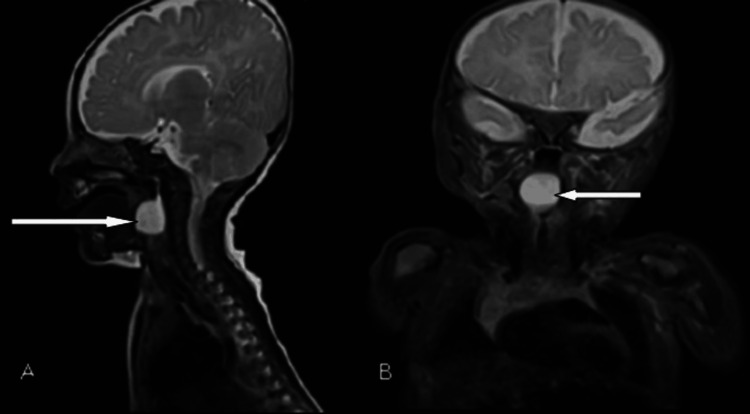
MRI sagittal (A) and coronal (B) section showing a well-defined rounded cystic lesion measuring 16×14 mm with broad base attachment abutting the posterior pharyngeal wall causing the almost complete airway obstruction (white arrow) MRI: magnetic resonance imaging
